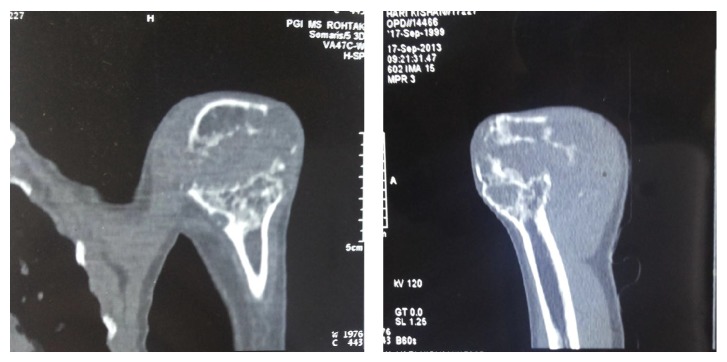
Preoperative CT showing exuberant new bone formation at the site of the deformity.
